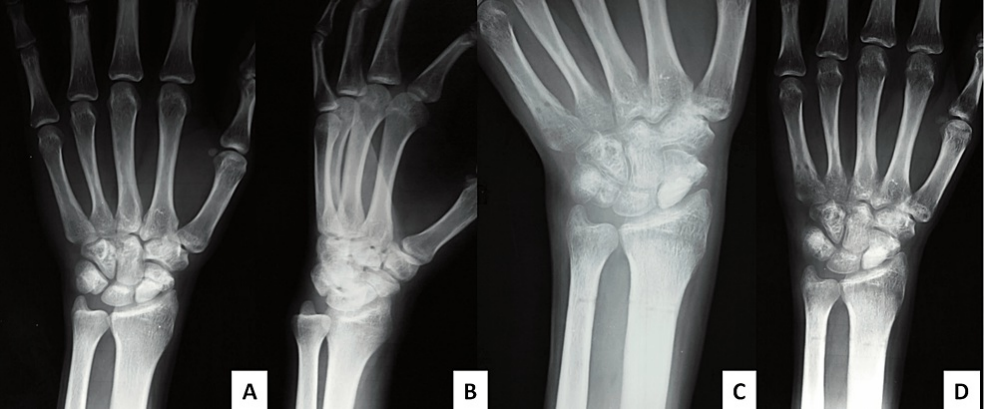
SNU in patient number 1, a preoperative radiograph (A,B) and a postoperative X-ray of the healed SNU in patient number 1 (C,D). SNU, scaphoid nonunion
